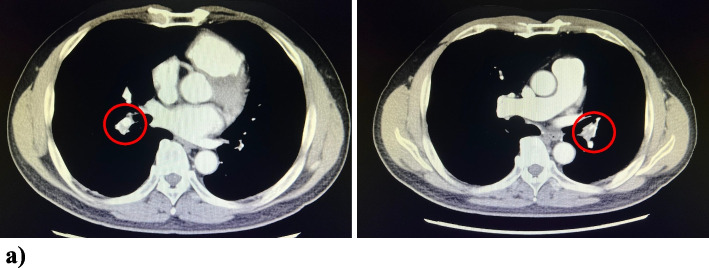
Thoracic contrast-enhanced computed tomography. a Pulmonary thrombi are found in the pulmonary arteries on both sides (red circle)
